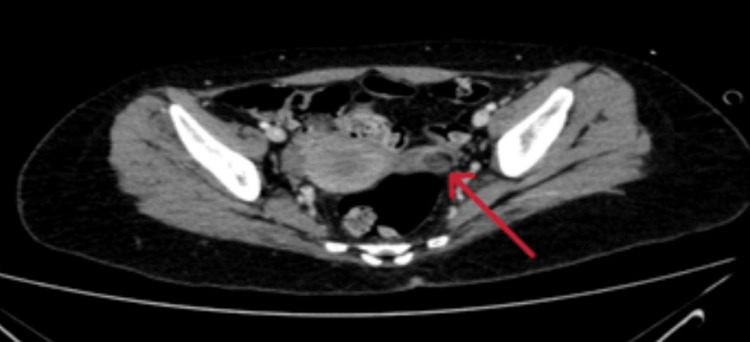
CT scan of the pelvis shows 11 mm solid cystic lesion on the left ovary
